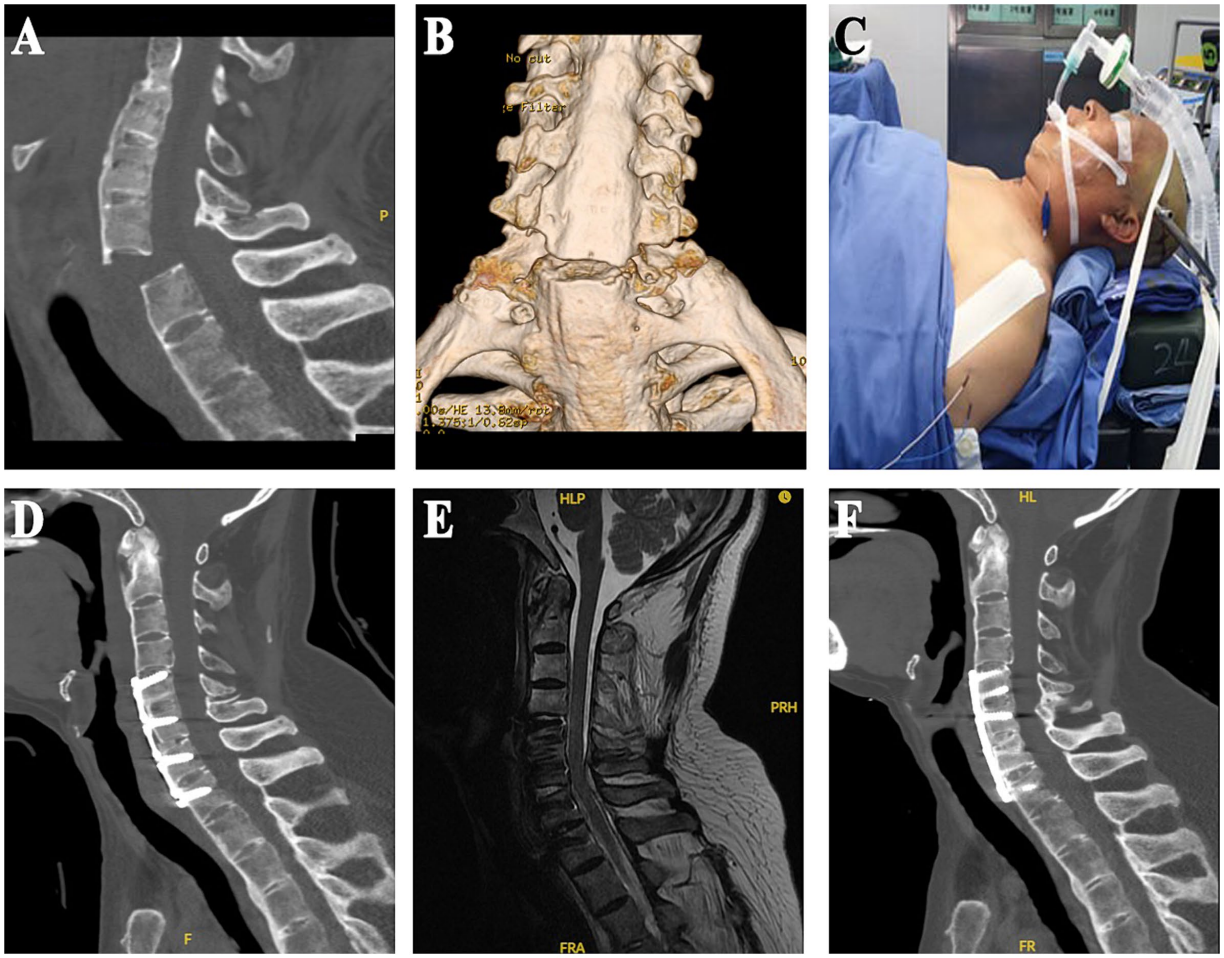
(A,B) Preoperative cervical spine CT sagittal and three-dimensional reconstruction images. (C) Special postures used by the patient during surgery. (D,E) Sagittal CT and MRI images within 1 week after surgery, CT indicated that the internal fixation position was good and stable, and the anatomical morphology of the spinal canal was restored to the maximum extent. (F) Sagittal CT images 6 months after surgery.
